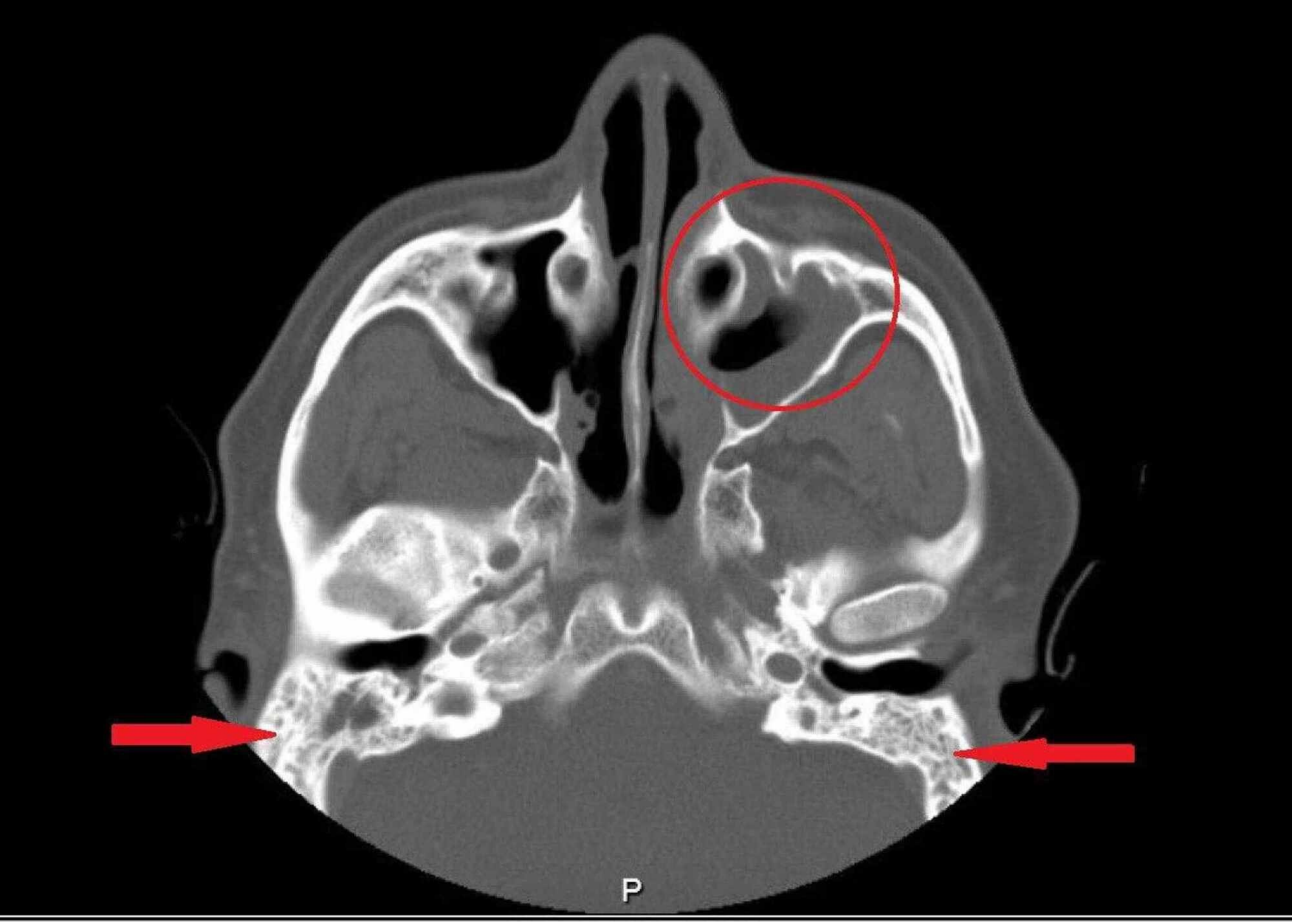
Paranasal sinuses computed tomography demonstrated bilateral otomastoiditis with interval development of severe left maxillary sinus mucosal disease.
